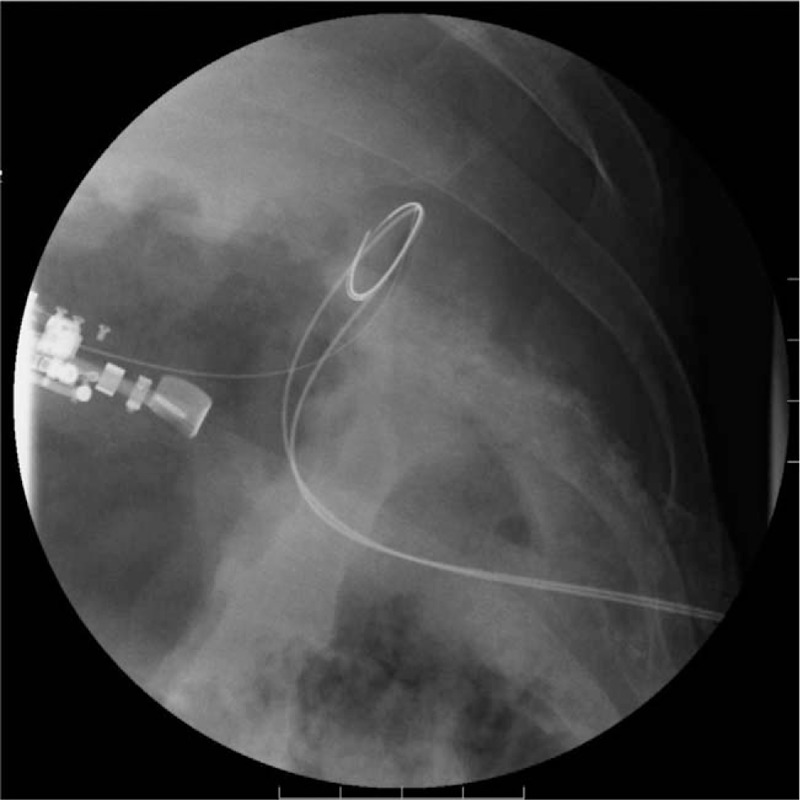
Fluoroscopic image. Guidewire insertion through the endoscopic ultrasound scope into the fistula cavity.
